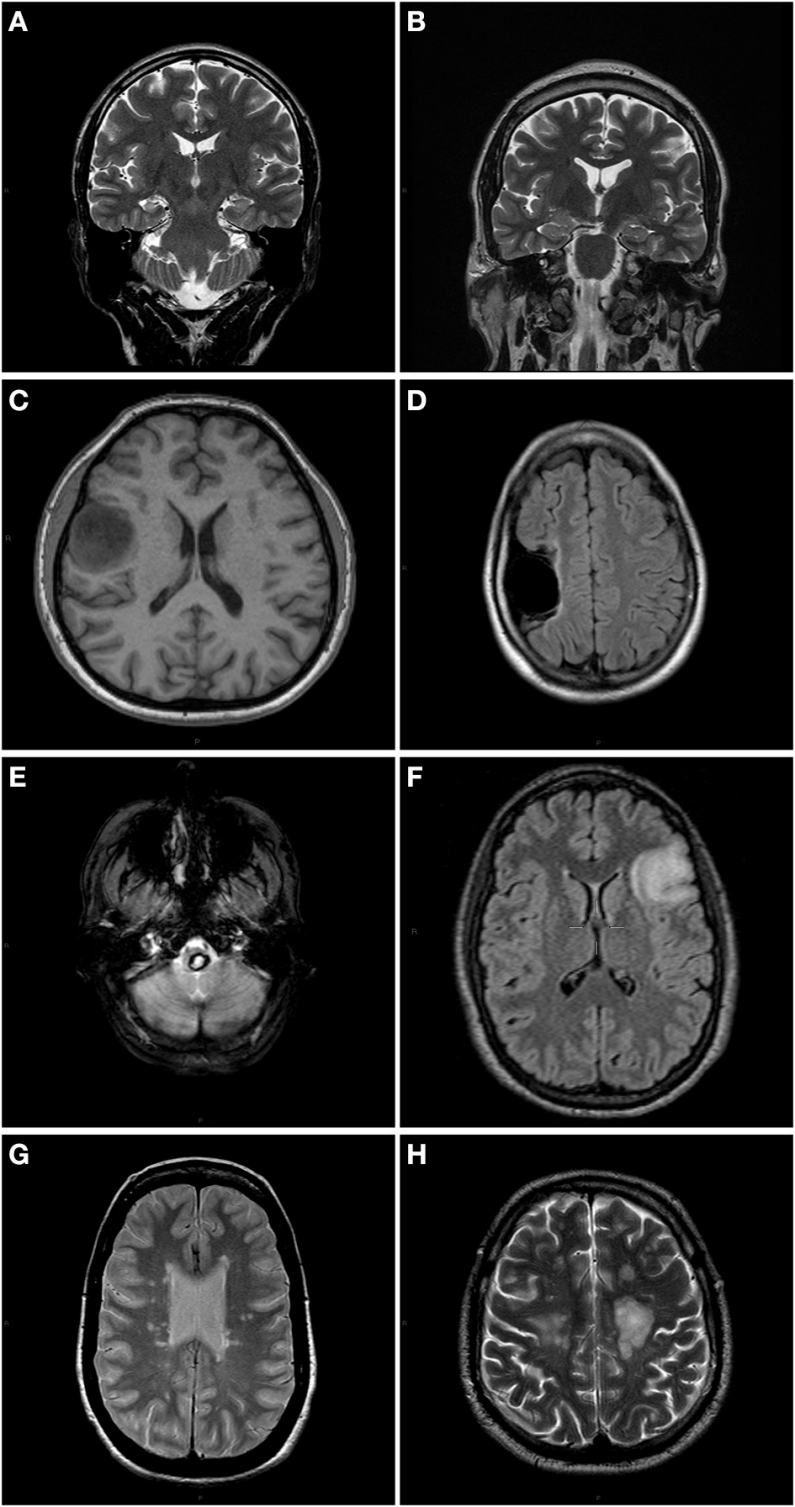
Anatomical slices of each patient showing different type of lesions. (A) P1: T2 weighted coronal slice. The right hippocampus volume is reduced. (B) P2: T2 weighted coronal slice. Slight decrease in right hippocampus volume. (C) P3: T1 weighted axial slice showing right precentral tumor. (D) P4: T2- FLAIR axial image showing a right temporal porencephalic cyst. (E) P5: T2 weighted axial slice of a patient with a medulla oblongata cavernoma in brainstem. (F) P6: T2-FLAIR of a left frontal glioma patient. (G) P7: PD axial slice of a MS patient. (H) P8: T2 weighted axial slice of a MS patient.
